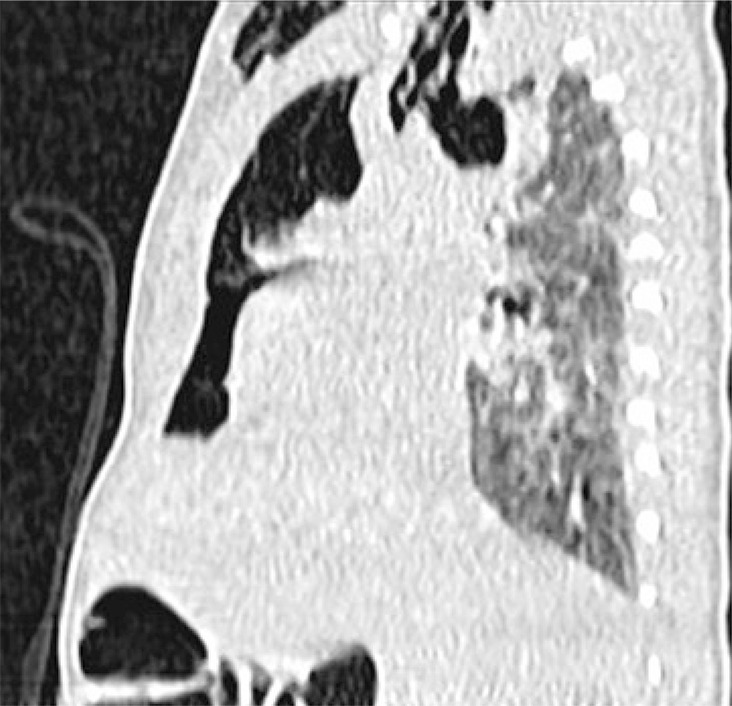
Computed tomography showing subcutaneous enphysema and extensive pneumomediastinum with mild septation, unchanged lung parenchyma (sagittal cut)
